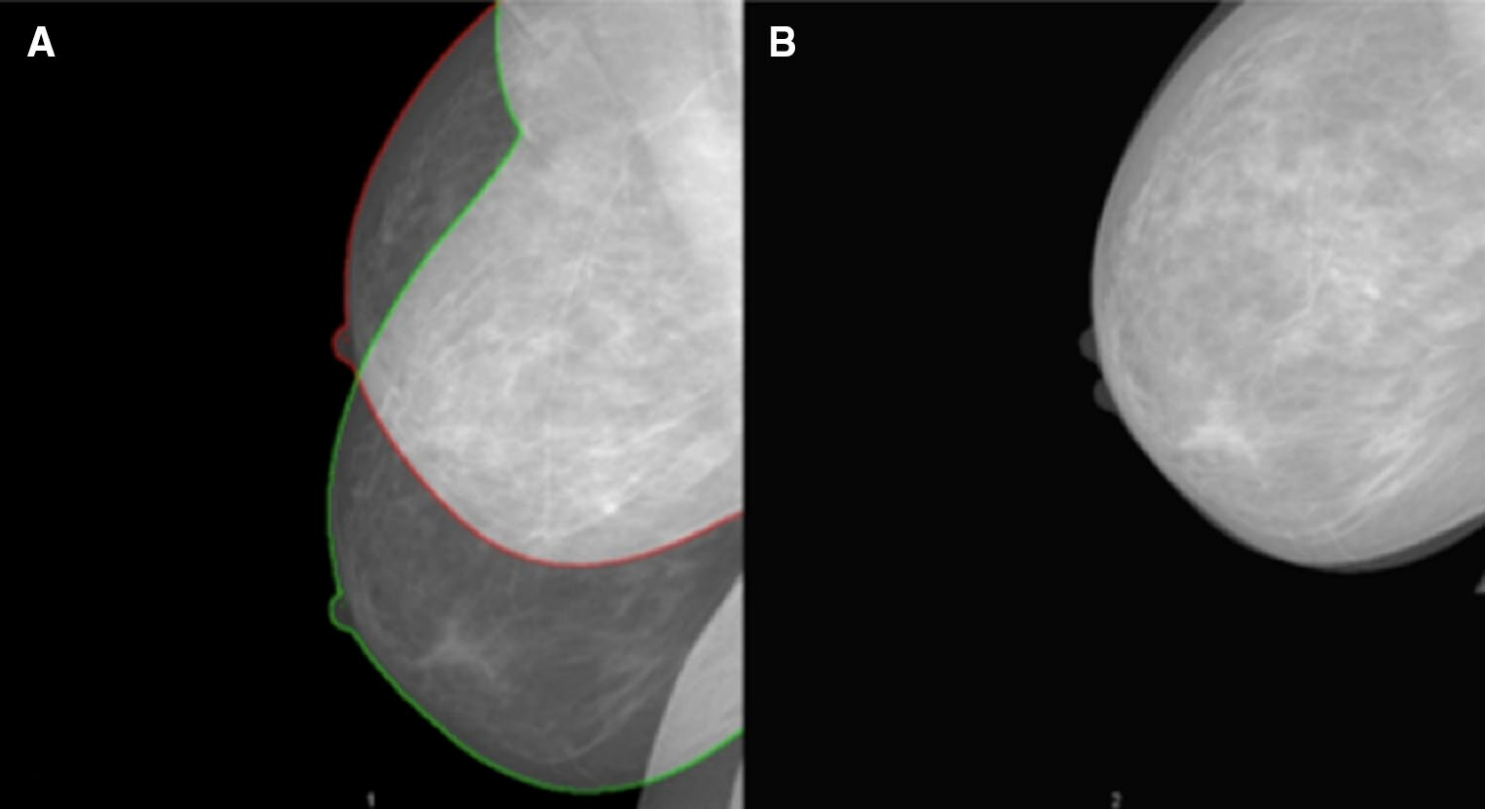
Two mammograms of the same breast were taken 2 min apart by the same radiographer. In Frame a, the mammograms were superimposed to show the difference in breast placement in the mammography machine. In Frame b, the two images were digitally aligned to the image showing the smallest breast size (outlined with red in Frame A) prior to density measurement
